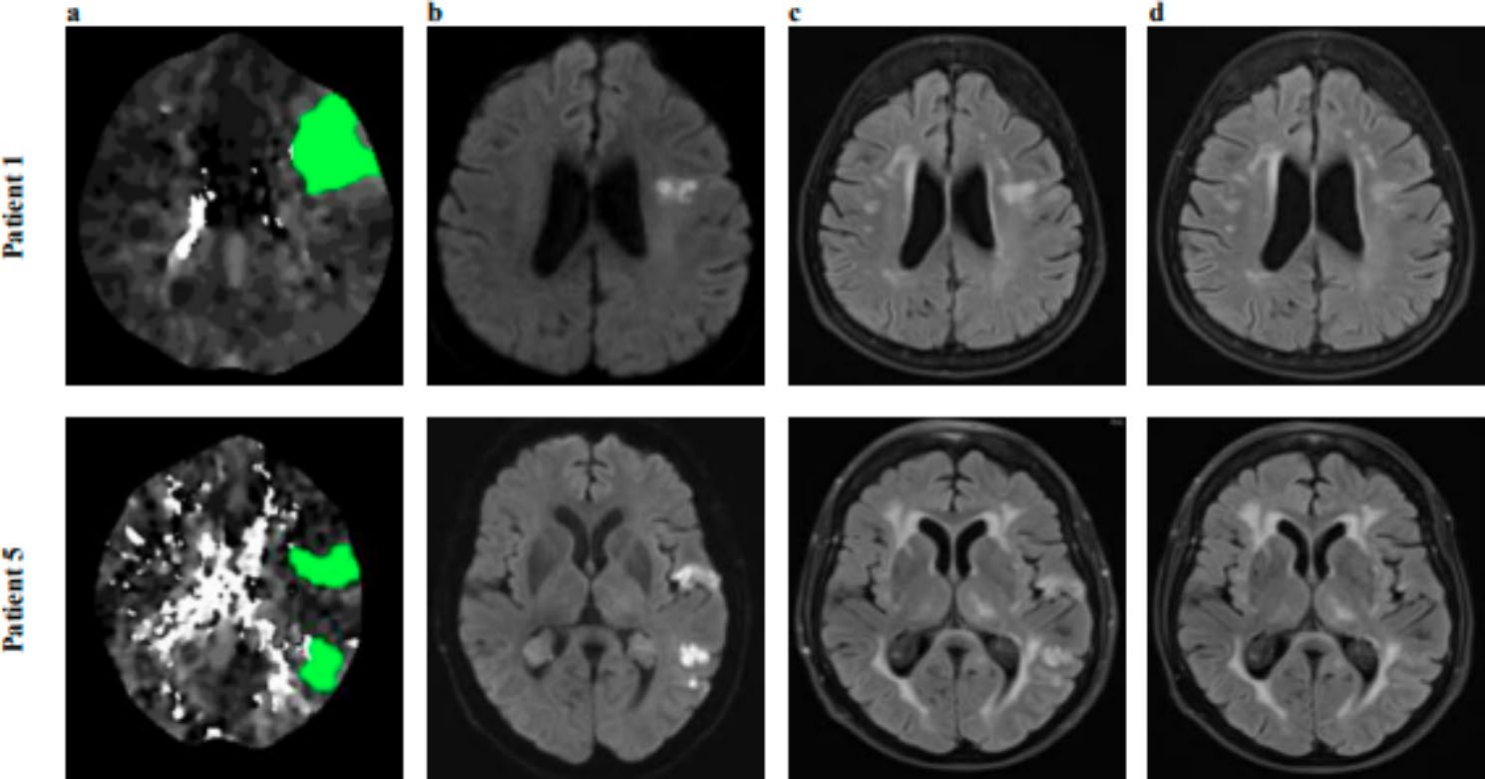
Tmax images on D0 (a) with relevant DWI (b) and FLAIR lesions on D3 (c) and FLAIR lesions on D9 (d) from patients 1 and 5
